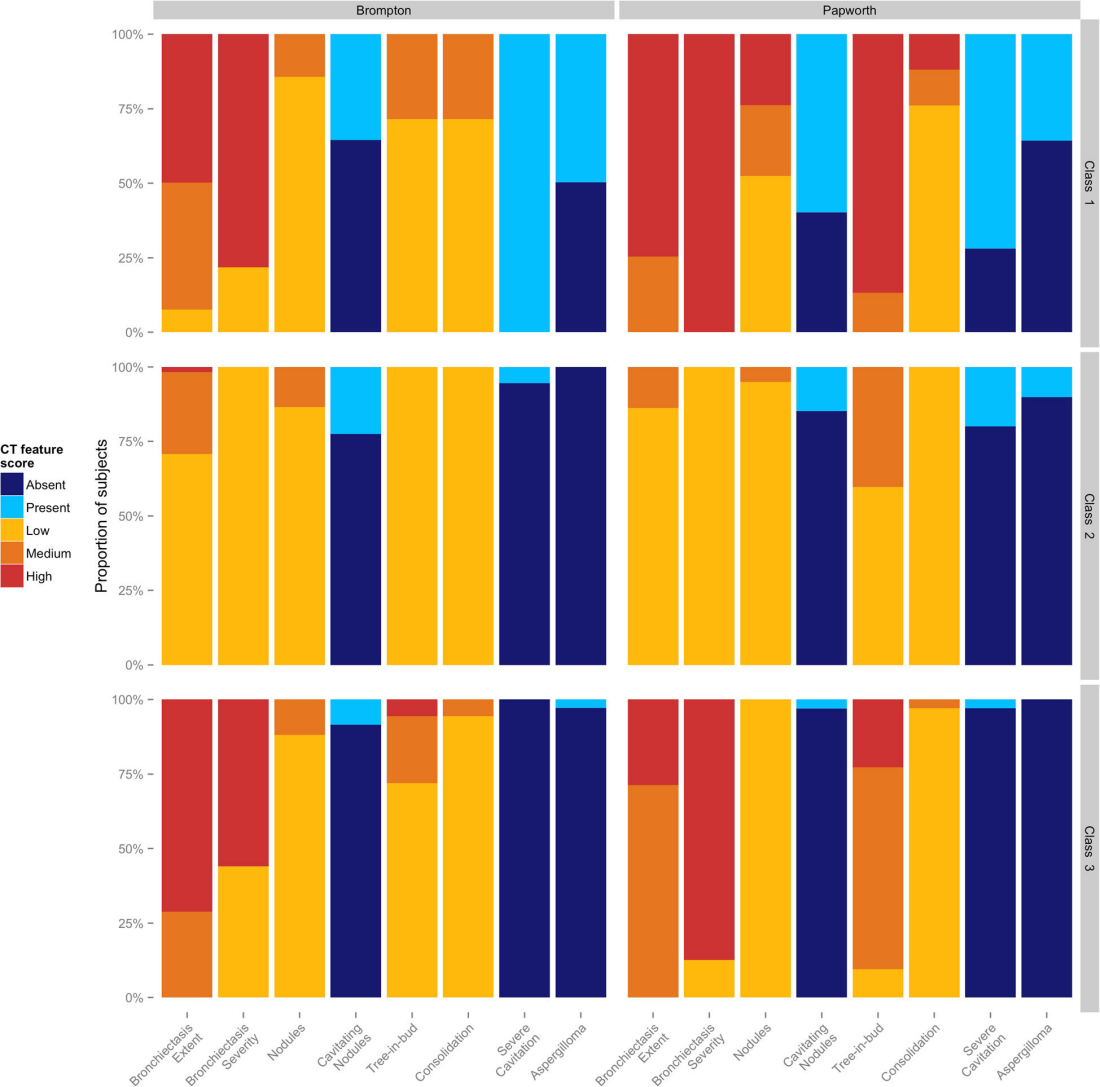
CT features of latent classes identified in the Royal Brompton (left panel) and Papworth (right panel) cohorts. The three classes identified by latent class analysis in each cohort are shown in the top, middle and bottom panels. The individual CT features used in the NTM scoring system are shown on the x-axes and the proportion of subjects on the y-axes. Colours represent the severity (low, medium or high), or the presence or absence of the CT feature
